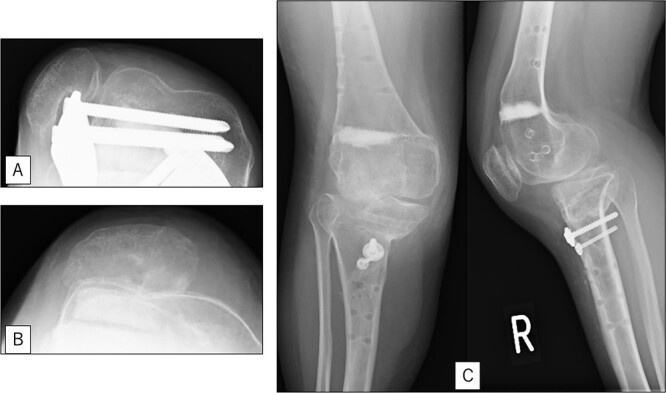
Radiography after right patellar re-dislocation and repair surgery: (A) the patella is eroded owing to contact with the femoral plate. (B) Skyline view after patellar repair surgery. (C) Anteroposterior and lateral view after patellar repair surgery.
